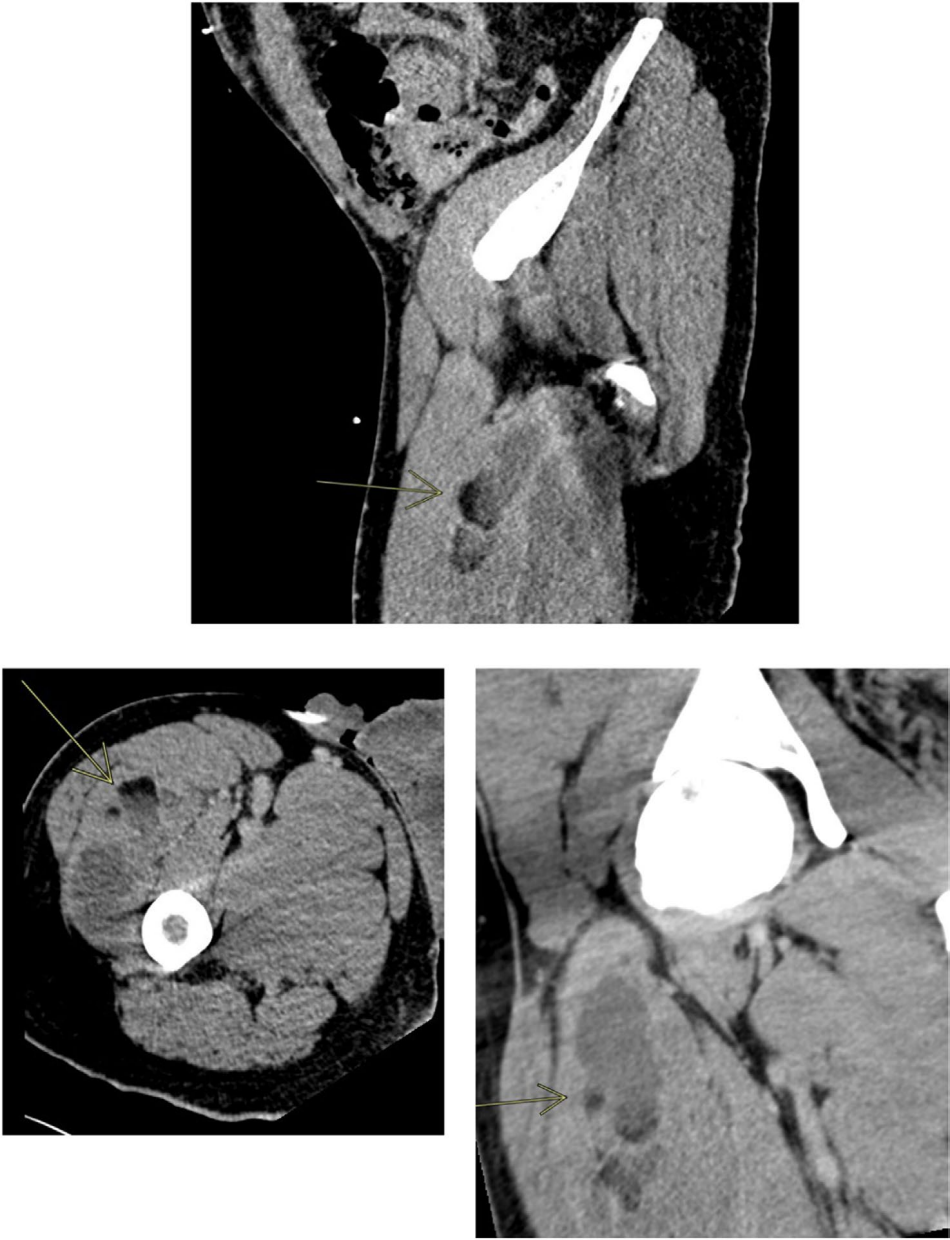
CT scan demonstration incompletely depicted large intramuscular collection in the right vastus intermedius muscle.
